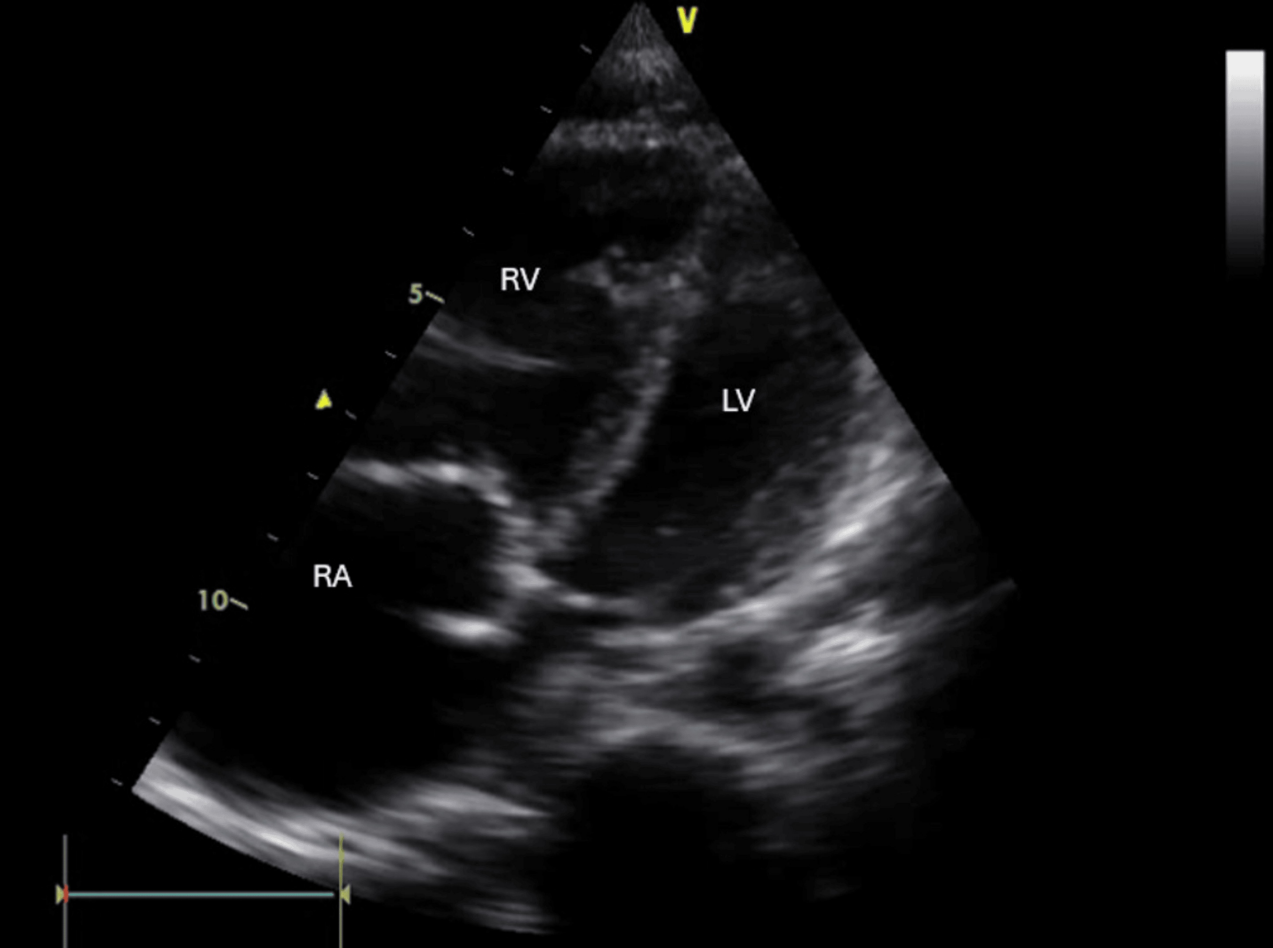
Echocardiography image with apical four chambers view shows normal LV dimensions and significantly dilated RV LV: left ventricle, RA: right atrium, RV: right ventricle.
